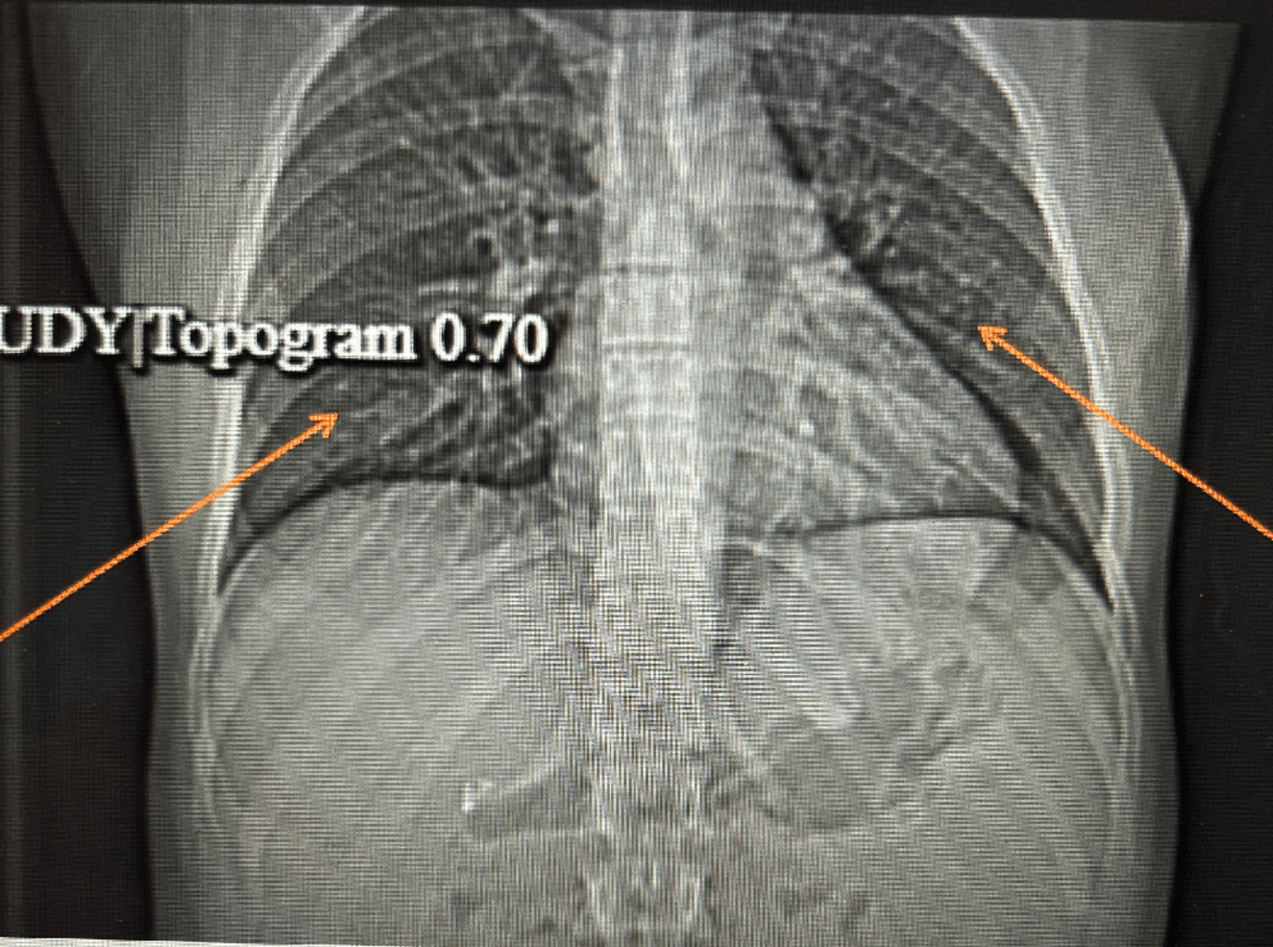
CT scan of the chest showing no evidence of pulmonary embolism.
